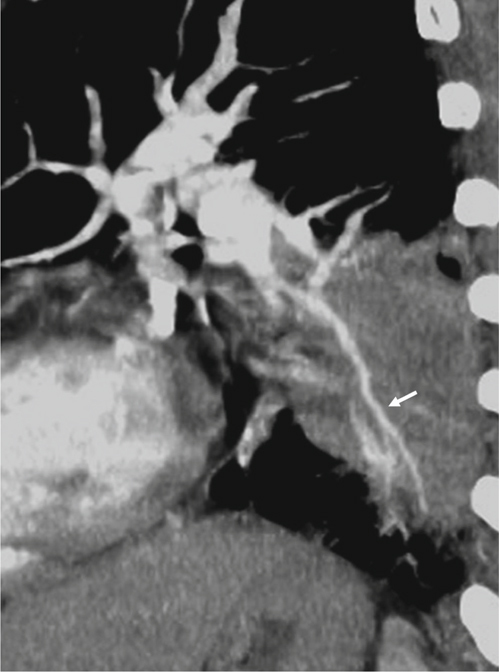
Penetration. This is a 64-year-old male with a mass lesion in the left lower lobe. He received ultrasonographyguided biopsy, and pathology revealed adenocarcinoma. Sagittal reformatted CT image reveals the pulmonary artery passes through the lesion without change of the vascular course or caliber of the pulmonary artery (white arrows).
